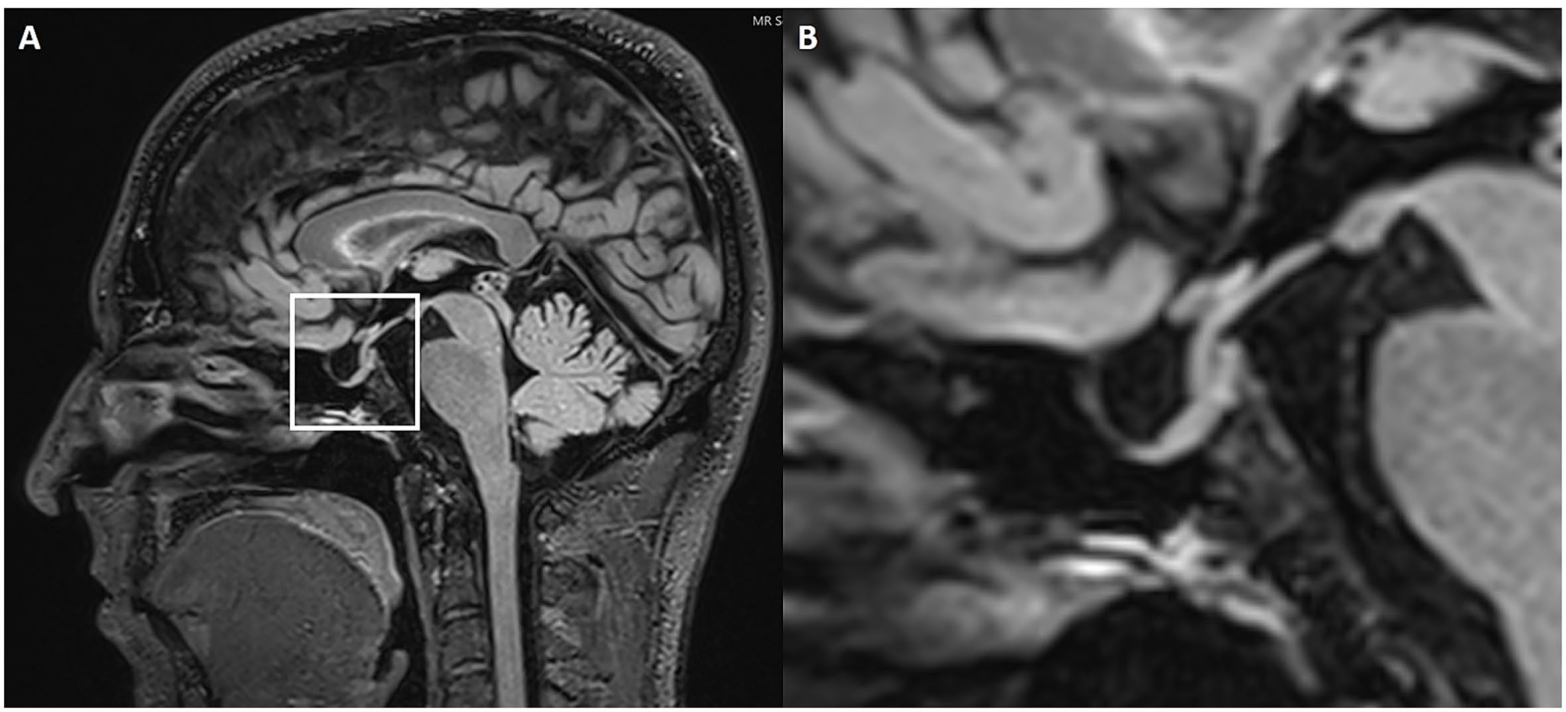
Partially empty sella. Sagittal 3D FLAIR (A) MRI with magnification (B). The white box highlights the sella turcica and pituitary gland. The magnified image shows a slight enlargement of the pituitary fossa, which is filled with CSF.
